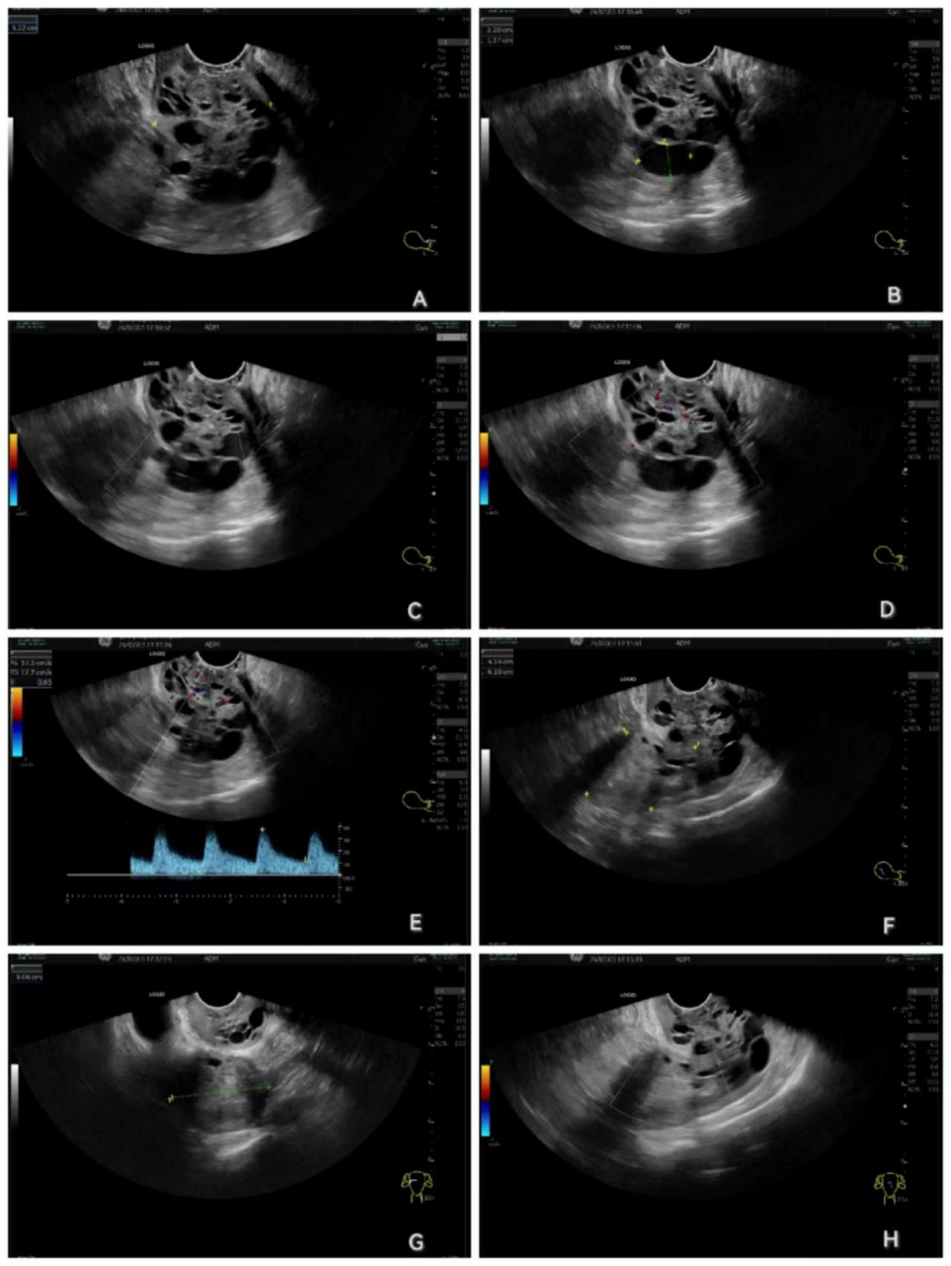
Transvaginal ultrasound images. (A) The cervix was thickened with an anteroposterior diameter of 4.3 cm and multiple cystic echoes. (B) The largest cystic echo of the cervix was approximately 2.2 cm x 1.4 cm. (C) Maximum cystic echoes of the cervix show no blood flow signal. (D) Punctate blood signals throughout the cervix. (E) RI: 0.66. (F) The anteroposterior diameter of the uterus was 4.3 cm with a longitudinal diameter of 6.1 cm. (G) The transverse diameter of the uterus was 5.1 cm. (H) The endometrium is poorly visualized without a blood flow signal but with the arterioles in the surrounding area.
